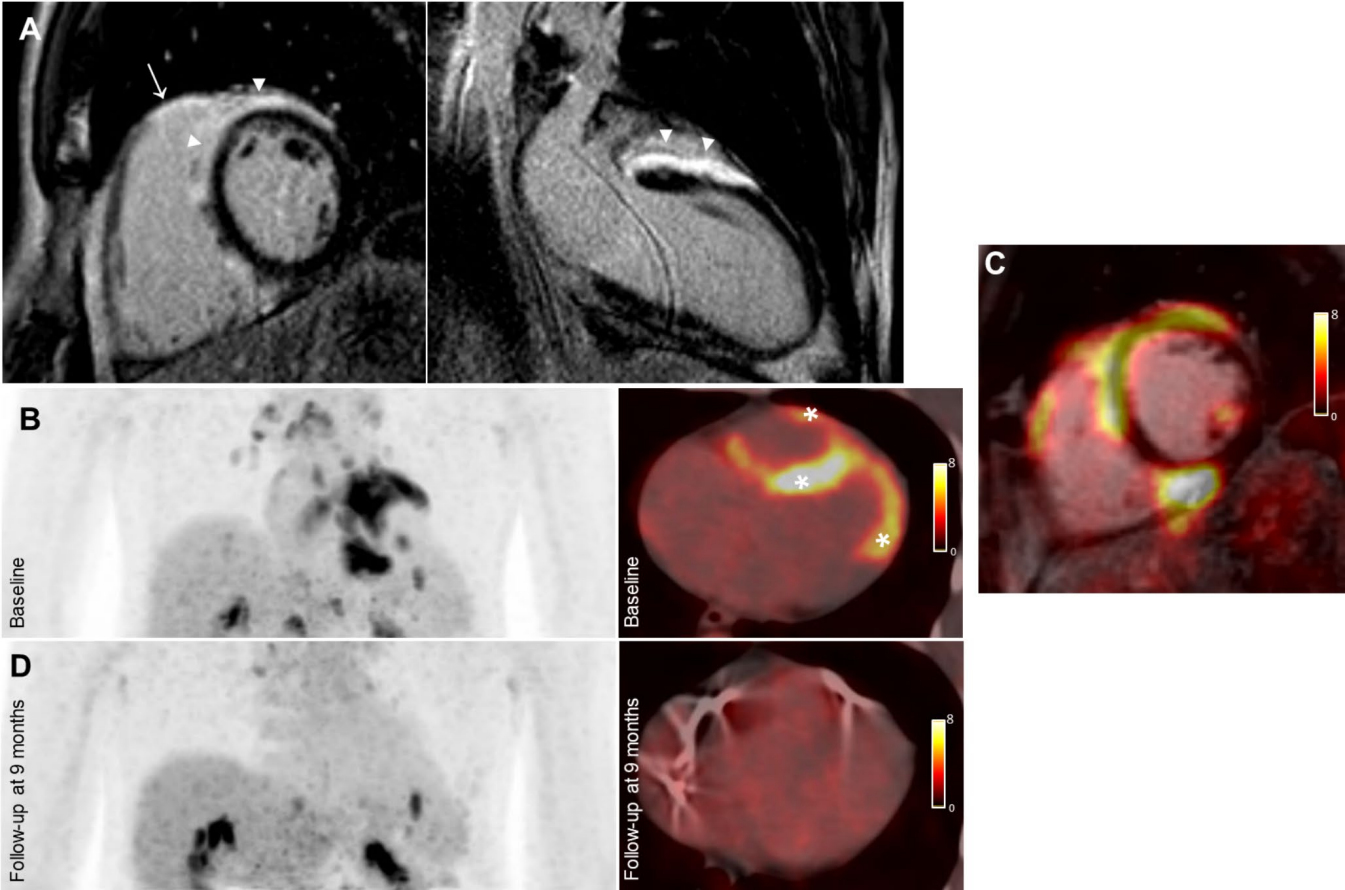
Advanced imaging. *A*: Cardiac MRI short axis and 2-chamber views, showing epicardial late gadolinium enhancement of the LV (anterior, anteroseptal and inferoseptal – arrowheads) and the RV free wall (arrow). *B*: Baseline ^18^F-FDG-PET/CT planar and axial images revealing multi-focal myocardial inflammation of the LV and the RV (asterisks). *C*: Axial fused image of cardiac MRI and baseline ^18^F-FDG-PET/CT, depicting matching of the epicardial fibrosis with the myocardial inflammation. *D*: Follow-up ^18^F-FDG-PET/CT, showing complete remission of the cardiac inflammation
